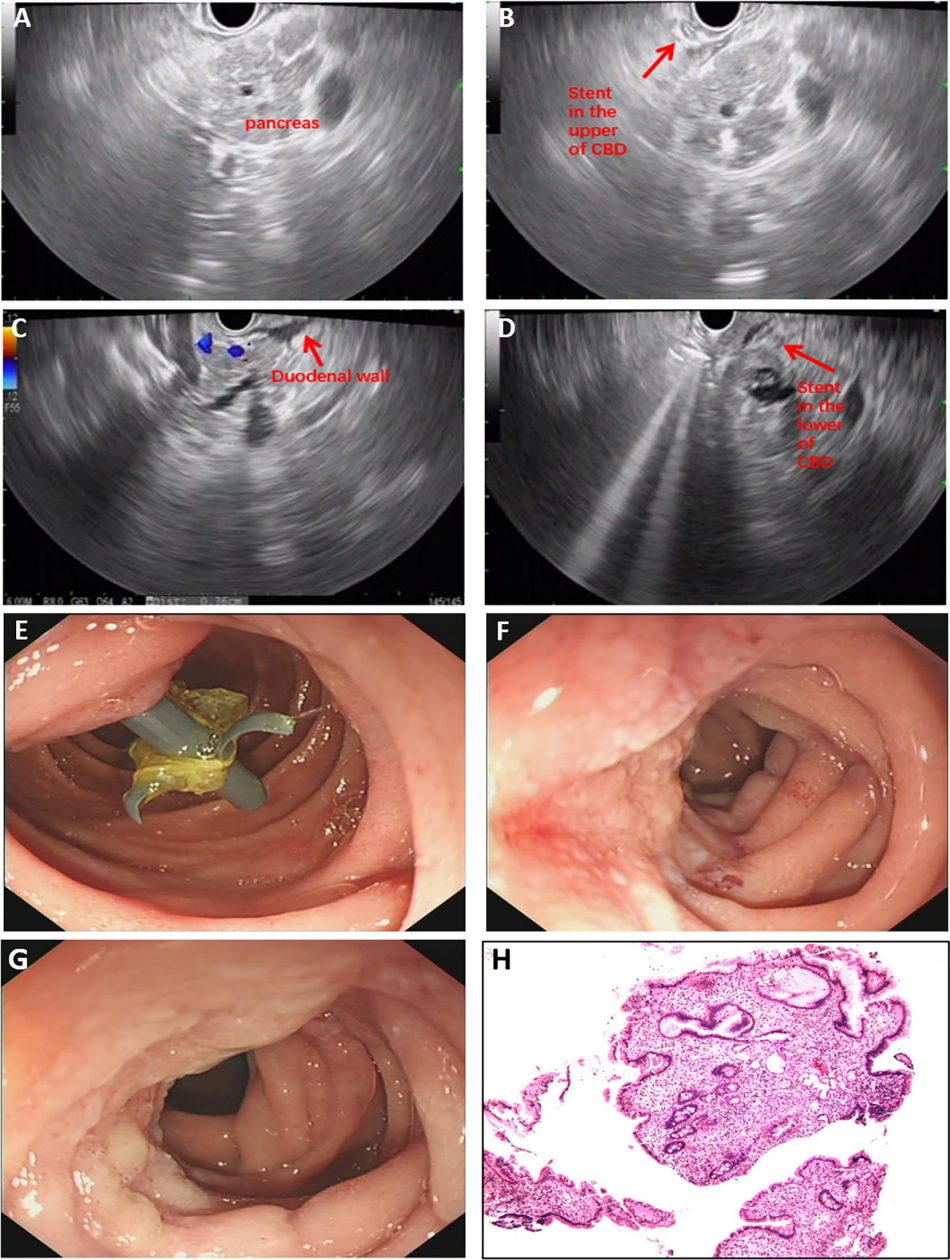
Examination results after admission. (A–D) The EUS scan showed the stent in the common bile duct and inhomogeneous echo of pancreatic parenchyma. (E–H) Gastroscopy found the rough mucosa of descending duodenum region and the remaining stent, but biopsy suggested inflammation. EUS, endoscopic ultrasonography.
